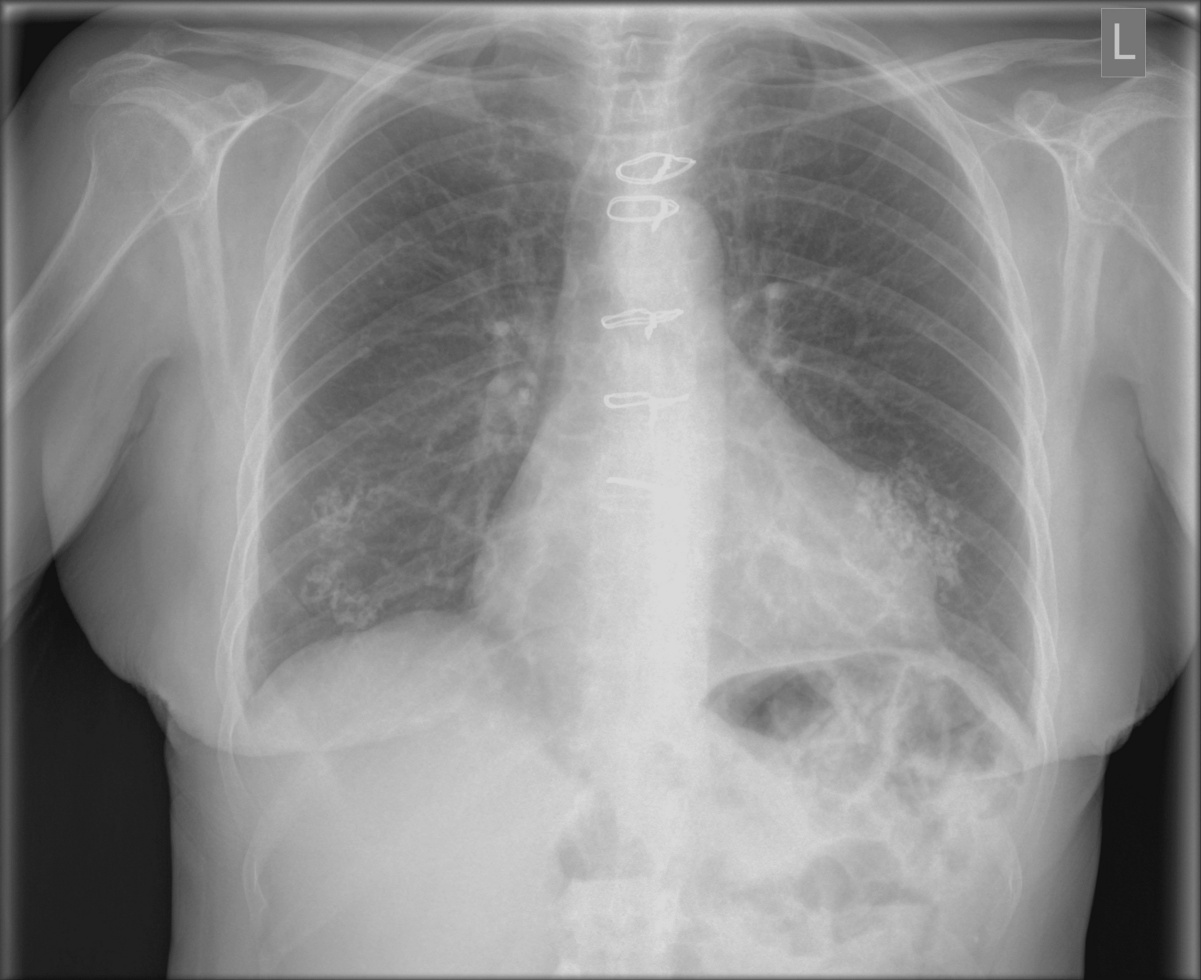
Erect chest X-ray showing no recurrence of hernia at six months postoperatively
